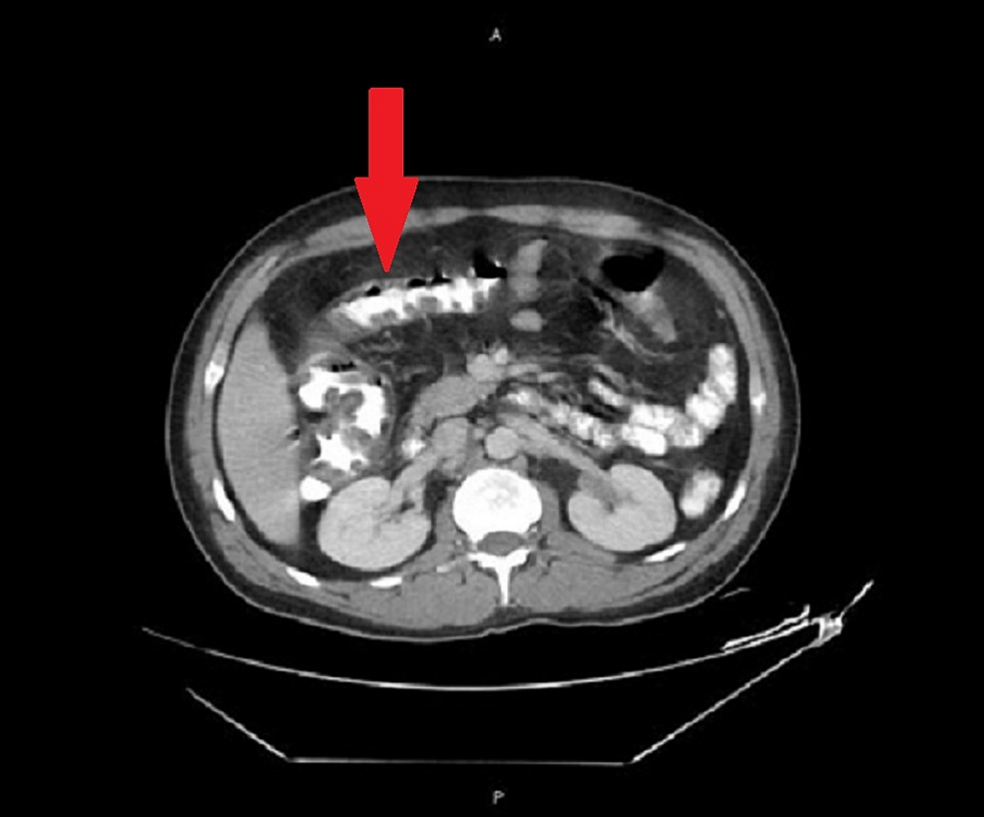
CT of the abdomen and pelvis with contrast showing diffuse colonic wall thickening from the rectosigmoid to the proximal ascending colon consistent with pancolitis.
